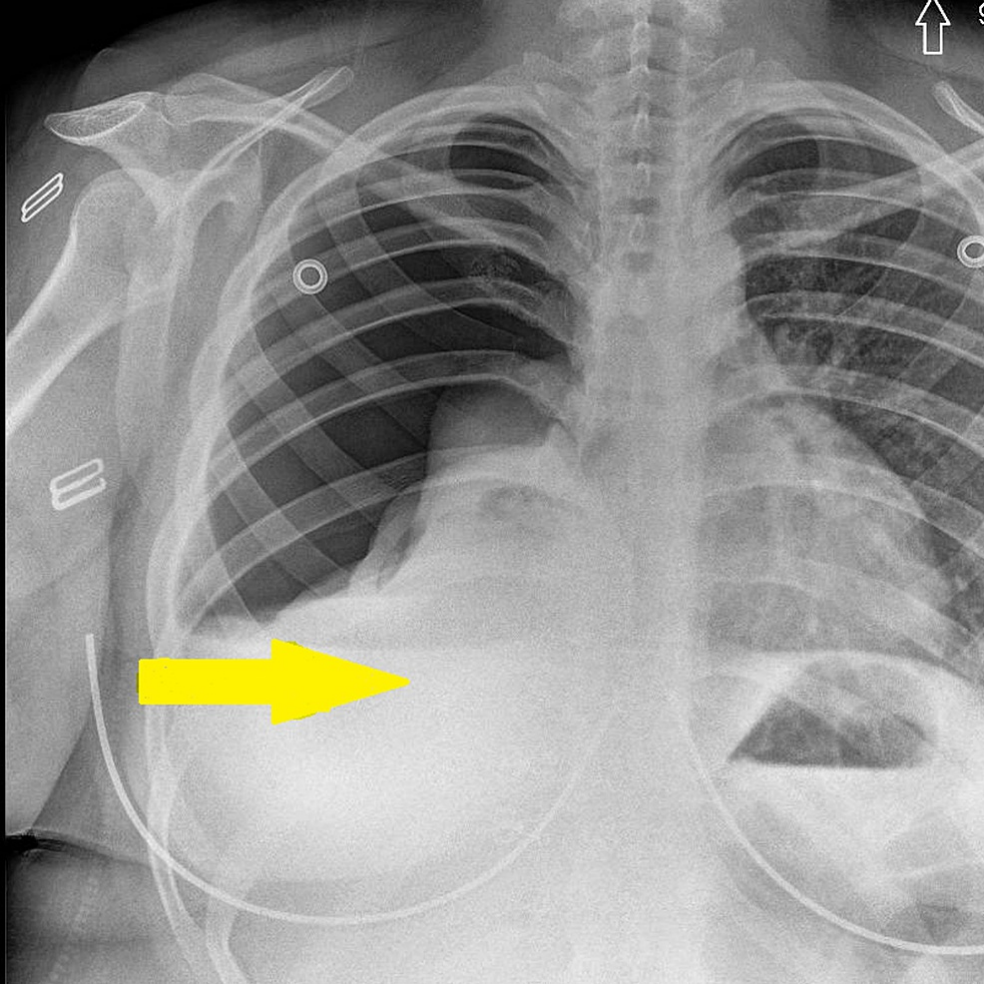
Chest x-ray (yellow arrow indicates lesion site).
